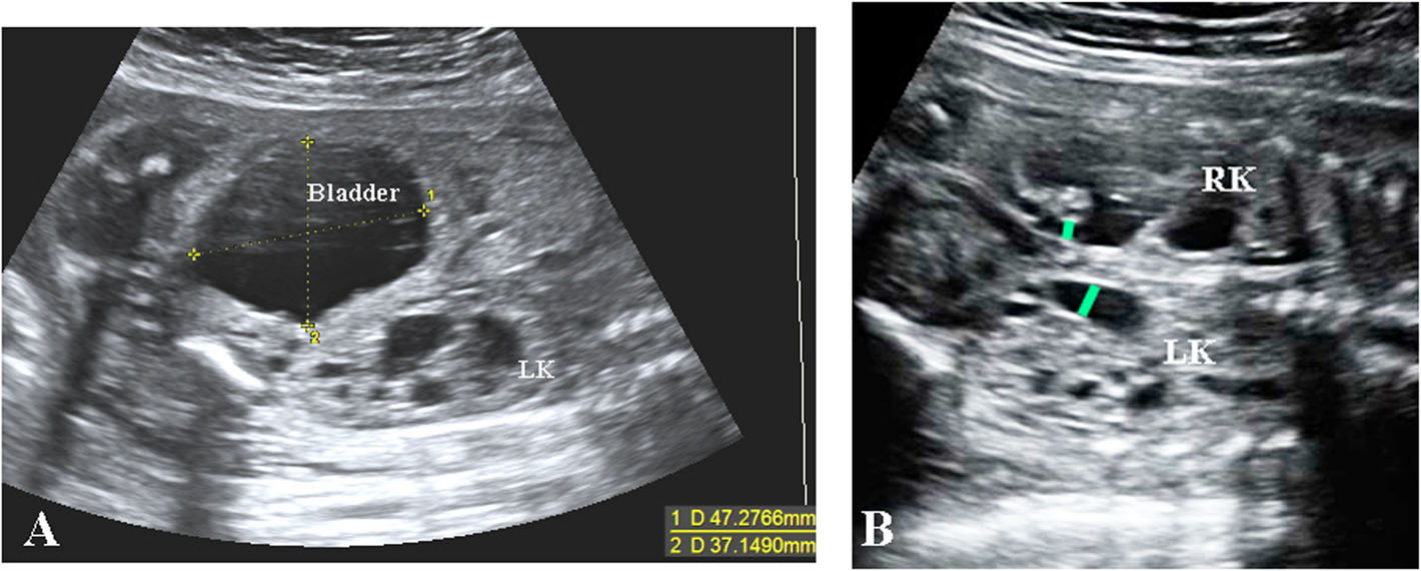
Ultrasonic features of fetal LUTO showed a distended fetal urinary bladder (a), fetal hydronephrosis and ureterectasis (b, green lines identify bilateral dilated ureters). RK, right kidney; LK, left kidney
